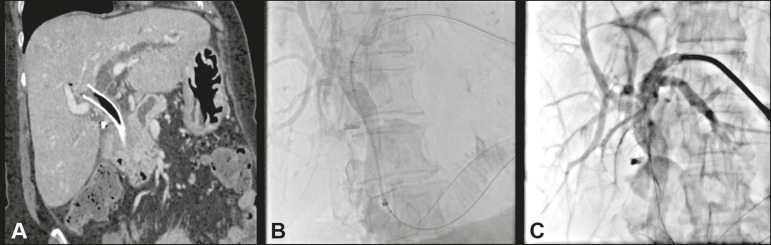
An 80-year-old female patient, with locally advanced adenocarcinoma of the pancreatic head, who presented with cholangitis. She underwent an endoscopic procedure to implant a metal stent (WallFlex; Boston Scientific, Marlborough, MA, USA). At two weeks after the procedure, her jaundice worsened. Computed tomography of the abdomen revealed marked dilatation of the left intrahepatic biliary tract-the consequence of an improperly positioned biliary stent, selective in the right hepatic duct by upward migration, occluding the confluence with the left hepatic duct (A). Another endoscopic procedure was performed to remove or reposition the stent. After its distal end had been captured, it was possible to crack the ring and the coating of the stent without moving it. Cholangiography showed peri-stent contrast retention and positioning of a different metal stent (Viabil; W.L. Gore, Flagstaff, AZ, USA) parallel to the WallFlex stent (B). Postprocedure cholangiography showed satisfactory biliary drainage into the duodenum (C).
